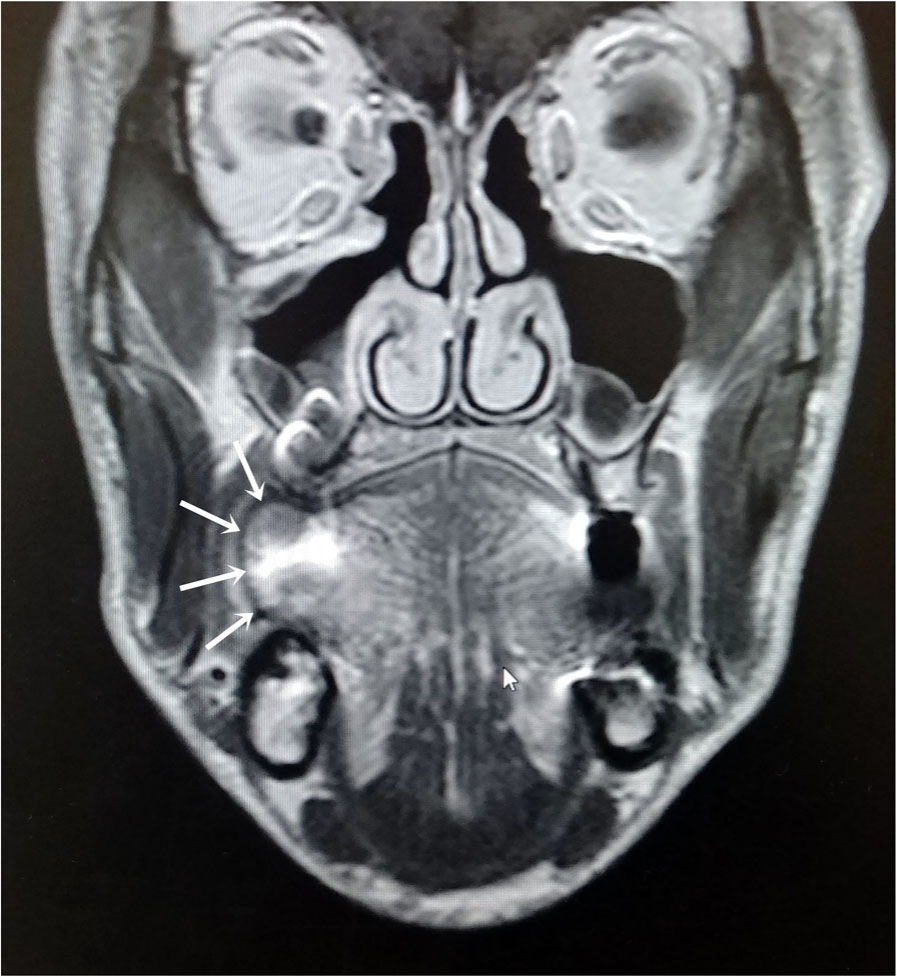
MRI showing a submucosal swelling within the muscles of the tongue on Rt. lateral side (white arrows)
